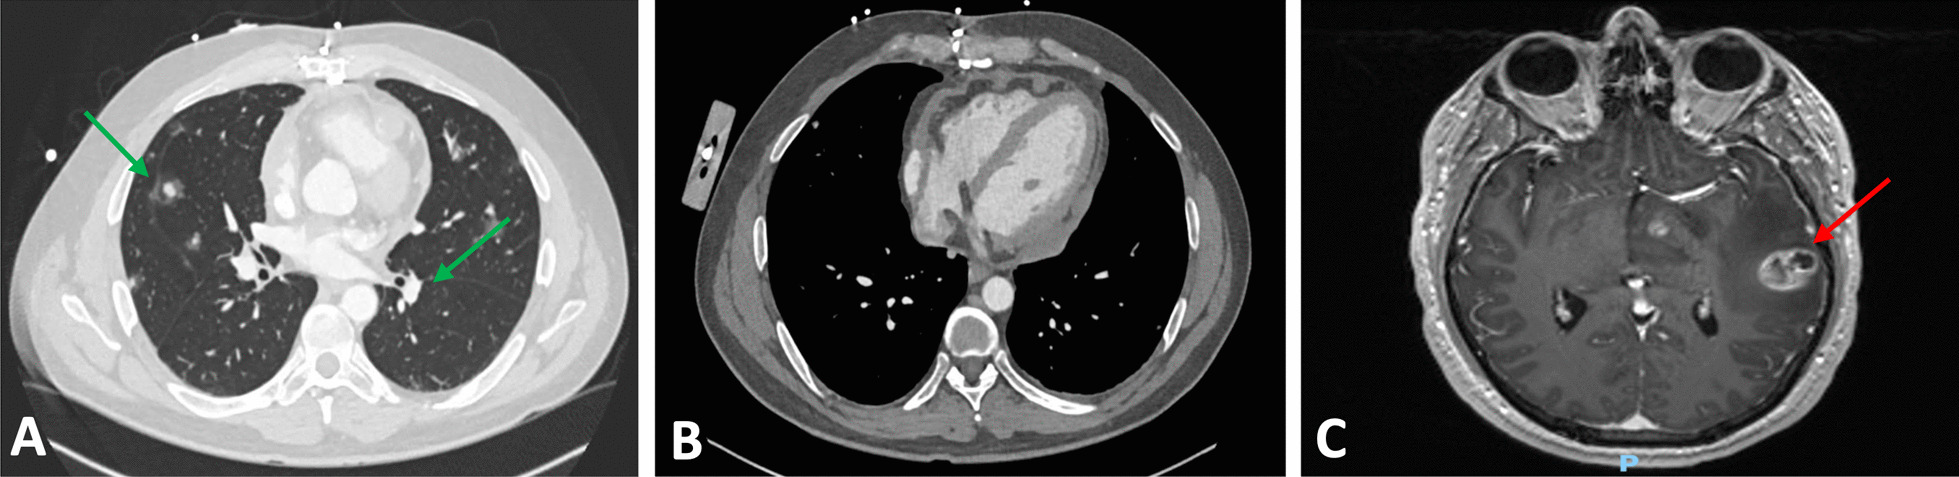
Imaging findings on representation. A Computed tomography (CT) of chest showing multiple lung nodules (green arrows). B CT of chest showing nodular appearance of pericardial effusion and outpouching of the right atrium. C Head magnetic resonance imaging (MRI) demonstrating peripherally enhancing lesions (red arrow)
